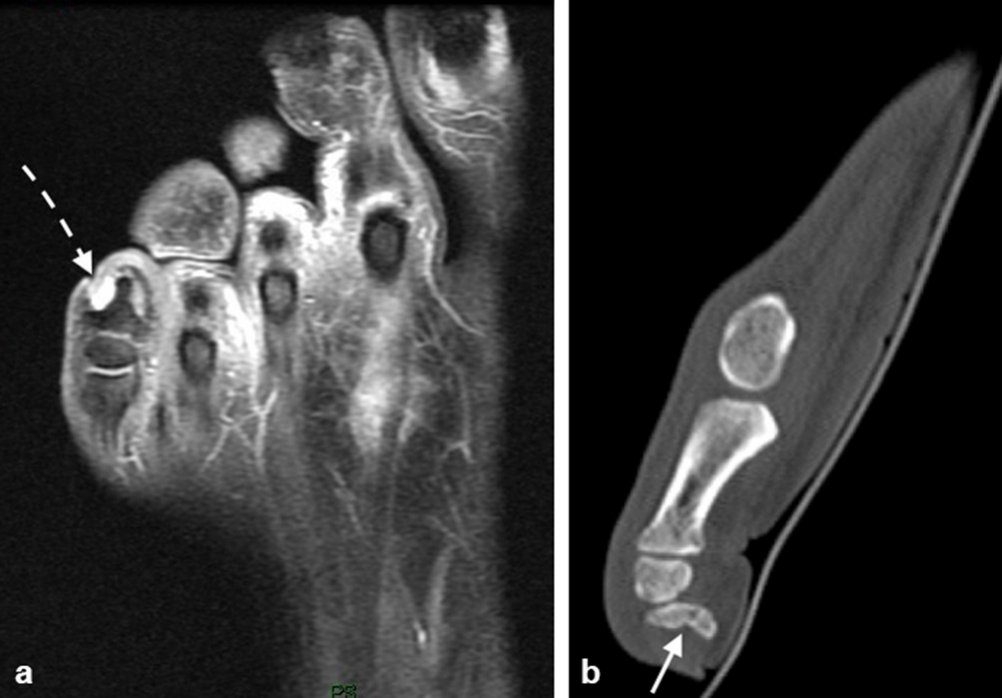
MRI axial post gadolinium fat suppressed *T*_1_ sequence (a) and sagittal reformatted CT (b) demonstrate resorptive remodelling of the distal phalanx of the fifth toe (arrow). Surrounding soft tissue enhancement (dashed arrow) is noted on the MRI.
